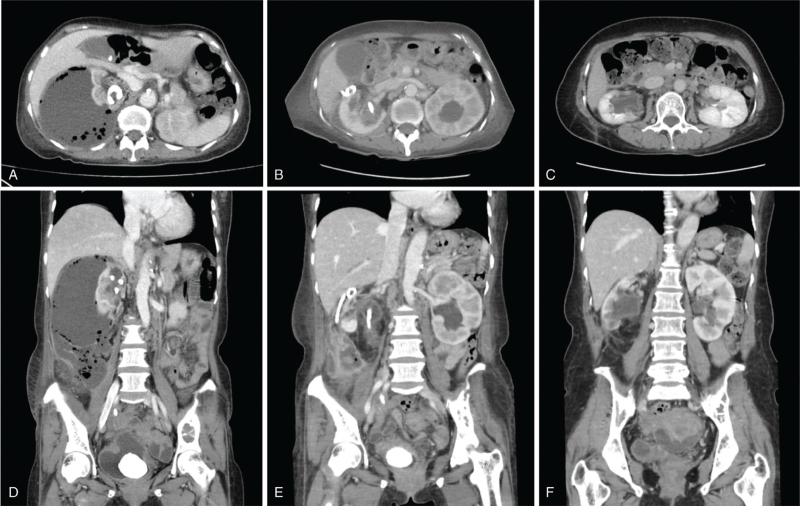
(A, D) Abdominal computed tomography (CT) scans on admission demonstrated a large perinephric abscess with gas formation and a forgotten double-J stent (DJS) in the right kidney. (B, E) Abdominal CT scans on hospital day 8 showed marked improvement of the right perinephric abscess after percutaneous catheter drainage. (C, F) Follow up abdominal CT two months after open ureterolithotomy revealed no significant inflammatory lesions. CT = computed tomography, DJS = double-J stent.
